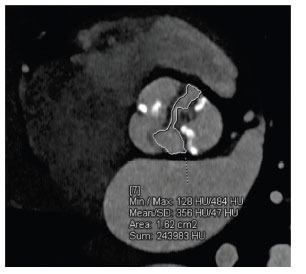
Axial contrast-enhanced computed tomographic angiography image showing the planimetry of the bicuspid aortic valve.
